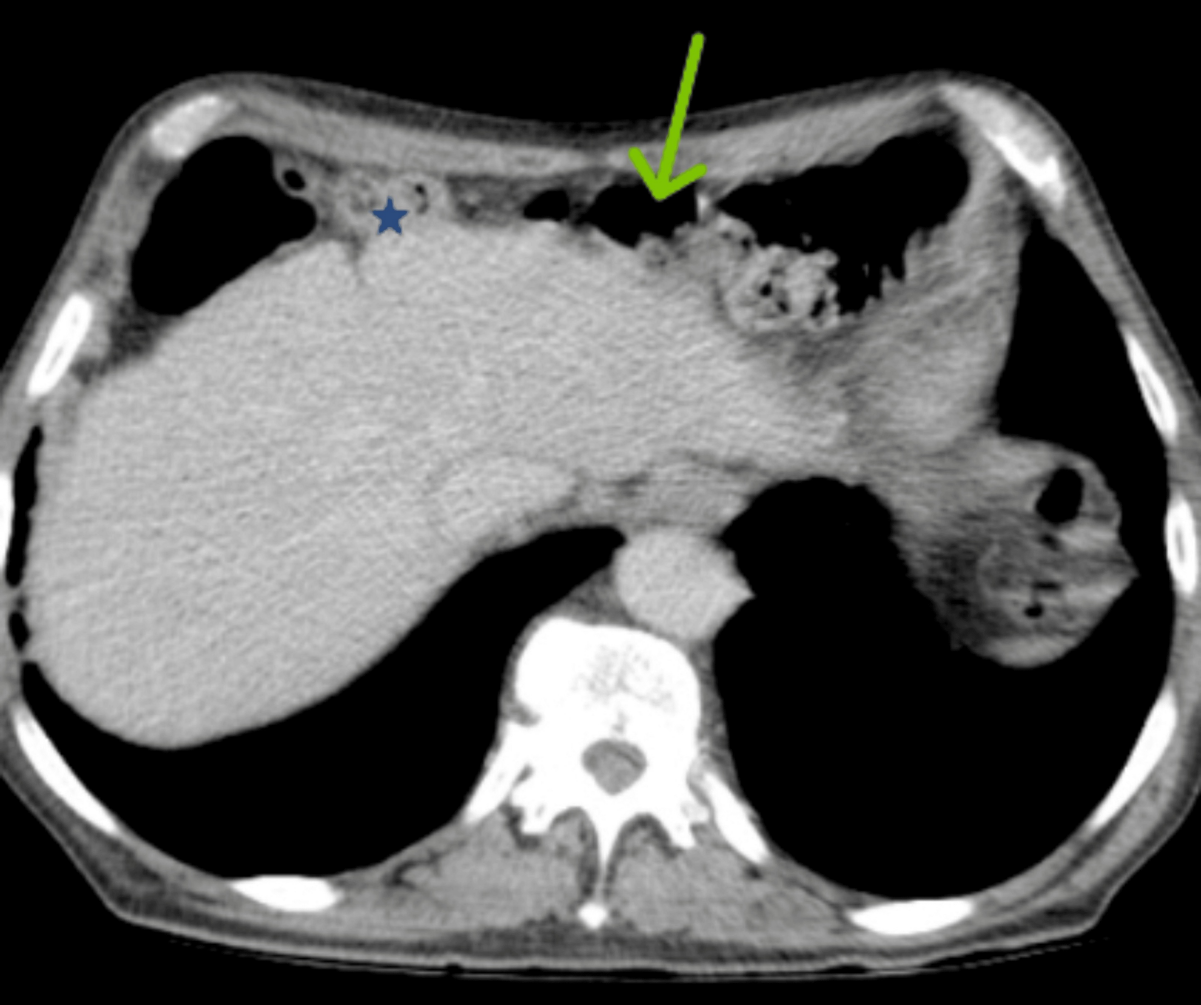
CECT image showing interposition of the ascending colon between the liver and the right hemidiaphragm, as denoted by the green arrow, a well-defined, heterogeneous mass lesion arising from the interpositioned ascending colon, characterized by central areas of necrosis and the presence of an air-fluid level within the lesion, as denoted by the blue star, consistent with the Torricelli-Bernoulli sign CECT: contrast-enhanced computed tomography
